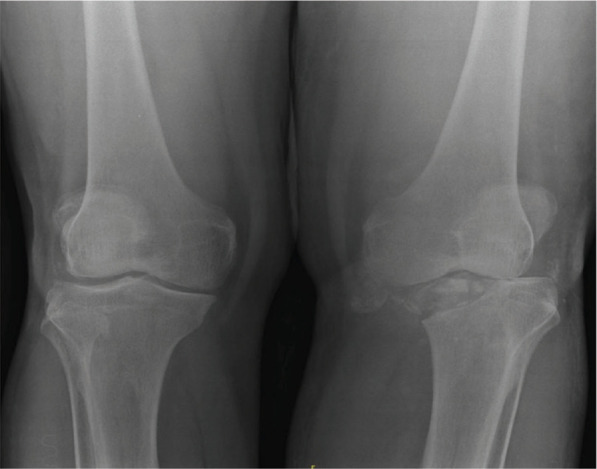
Radiographs AP view of both knees, on admission showing marked destruction of medial tibial plateau of the left knee. There is lateral subluxation of the tibia.
